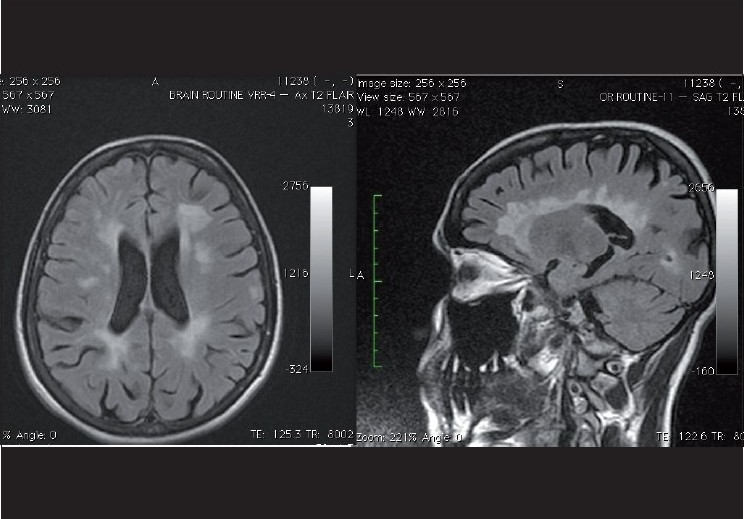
Left: Axial T2 FLAIR image at the level of the lateral venticles showing multiple hyperintense periventricular white lesions, suggestive of Multiple Sclerosis. Right: Para sagittal T2 FLAIR image showing multiple hyperintense periventricular white lesions perpendicular to the lateral ventricles, also known as Dawsons fingers
